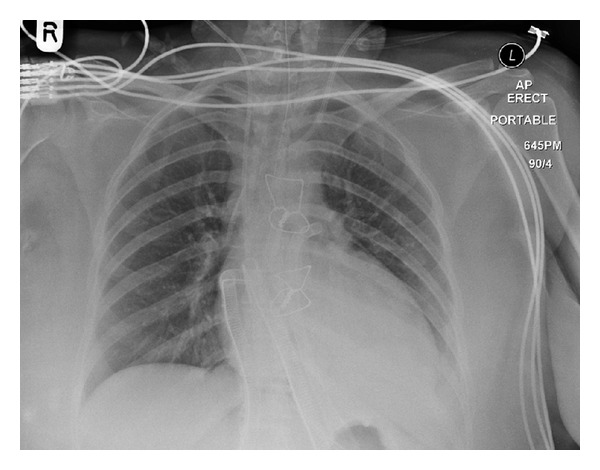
Chest X-ray (case one). Showing implanted BiVAD.
